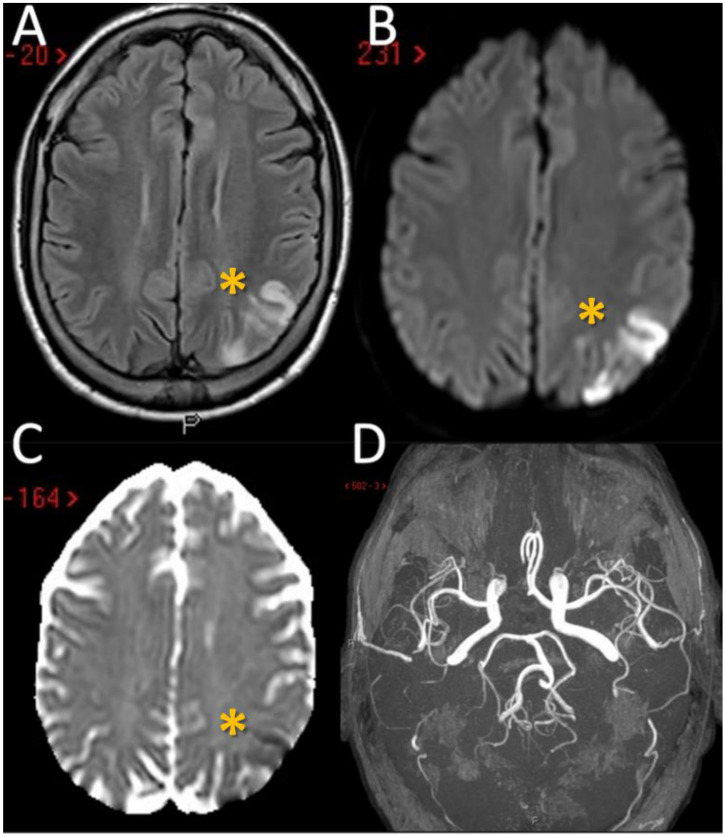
Brain MRI of the first patient. MRI of patient 1 showed the presence in the axial Fluid Attenuated Inversion Recovery (FLAIR) sequences of a wedge of cortical ischemic lesions in the left posterior parietal lobe (yellow asterisk) (A) with DWI and ADC sequences pattern suggesting acute ischemic lesions (B,C). MRA (time-of-flight [TOF] reconstruction) shows fully patent proximal intracranial arteries and right A1 anterior cerebral artery (ACA) aplasia (D). MRI: Magnetic Resonance Imaging; MRA: Magnetic Resonance Angiography; DWI: Diffusion Weighted; ADC: Apparent Diffusion Coefficient.
